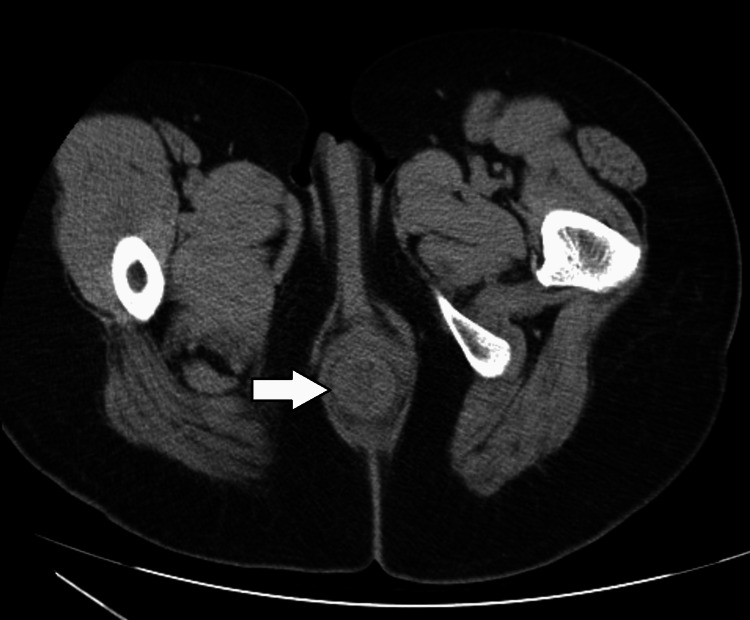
Computed tomography of the perianal region Computed tomography of the perianal region in the supine position revealed no obvious mass lesions in the rectum or anus, and rectal prolapse was suspected (arrow)
